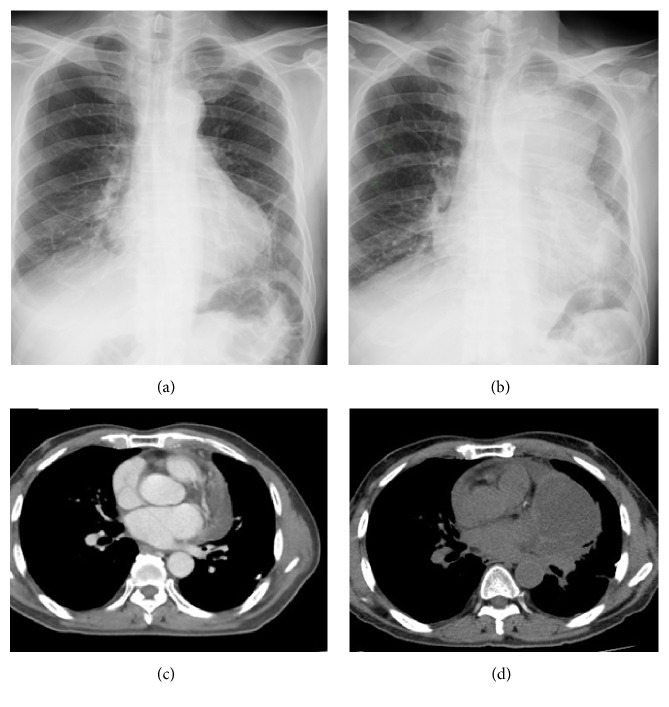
Central parenchymal pulmonary lesions. The patient is a 63-year-old man with renal cell carcinoma who underwent targeted therapy. He felt dyspnea. Chest X-rays were taken at time (a) and 1 month later (b), which shows the rapid progression of the left upper lung mass. A CT scan (c) shows the bulging left superior vein and 1 month later (d) reveals the lung parenchymal mass located outside the left superior pulmonary veins.
